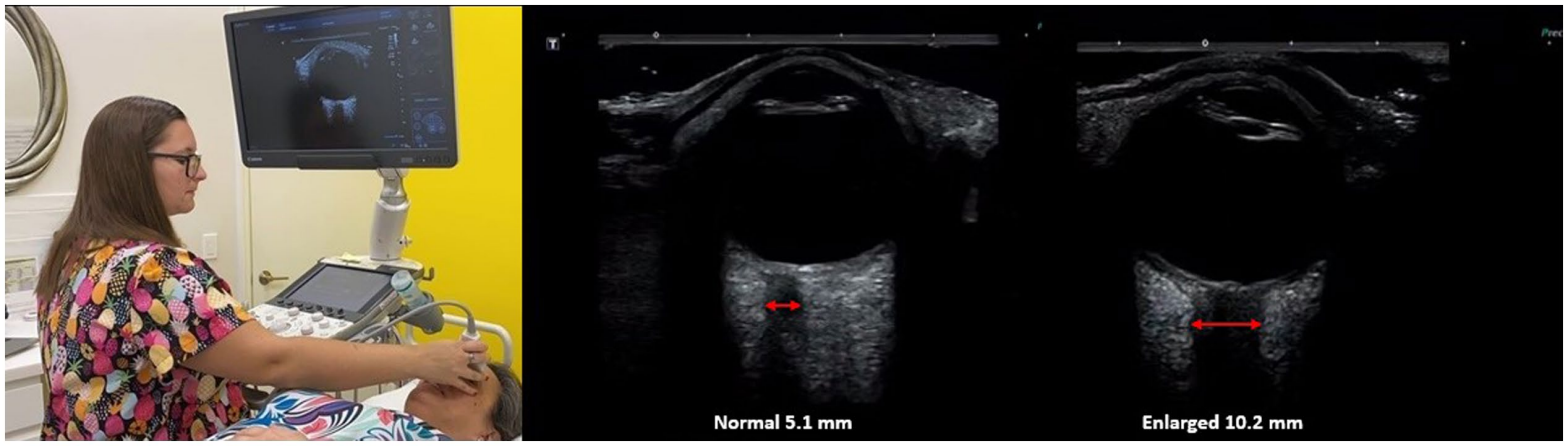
Ultrasound of eye to measure optic nerve sheath diameter, which is used as a marker for intracranial hypertension (increased brain pressure).
